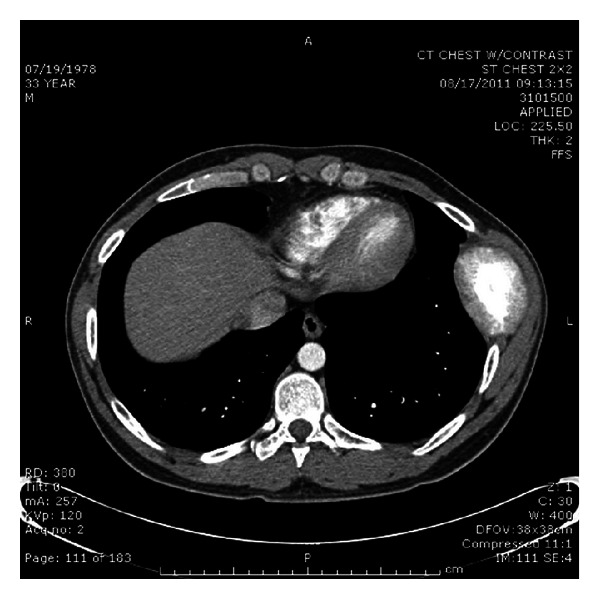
CT scan of the chest showing a large mass of the left lateral aspect of the seventh rib with bony matrix and sunburst central core.
